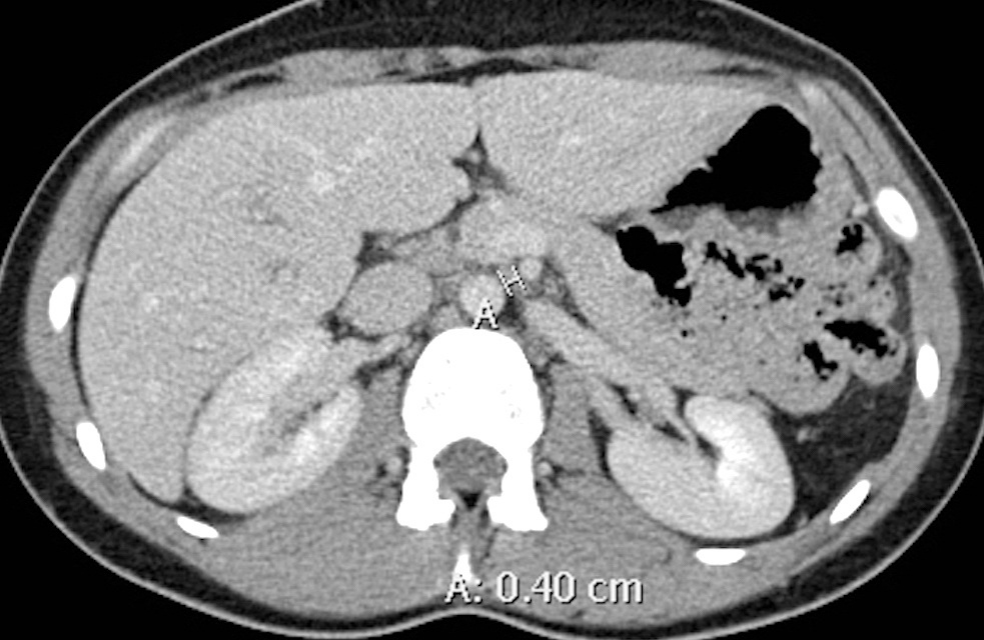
Contrast-enhanced axial CT image demonstrates a narrow aortomesenteric distance of 4 mm (calipers) (normal > 8 mm).
